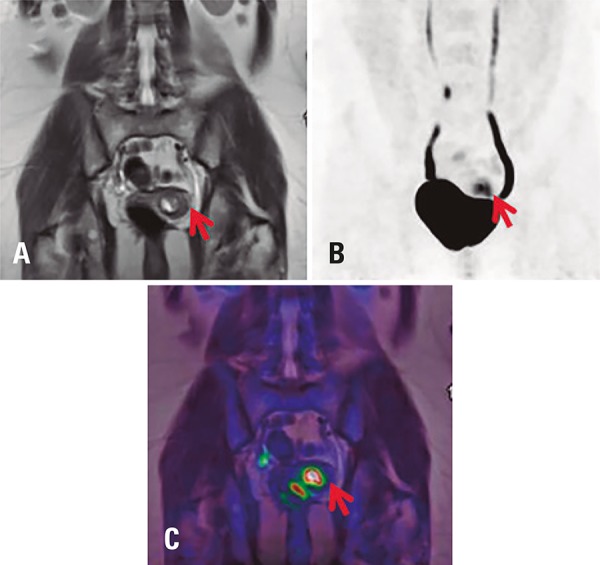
18F-FDG PET/MRI images of a female pelvis with invasive adenocarcinoma of the cervix showing metabolic activity in the left uterine wall, near the uterine cavity. (A) Dixon VIBE, coronal T2; (B) PET, maximum intensity projection image; (C) fused PET/MRI image, coronal T2
